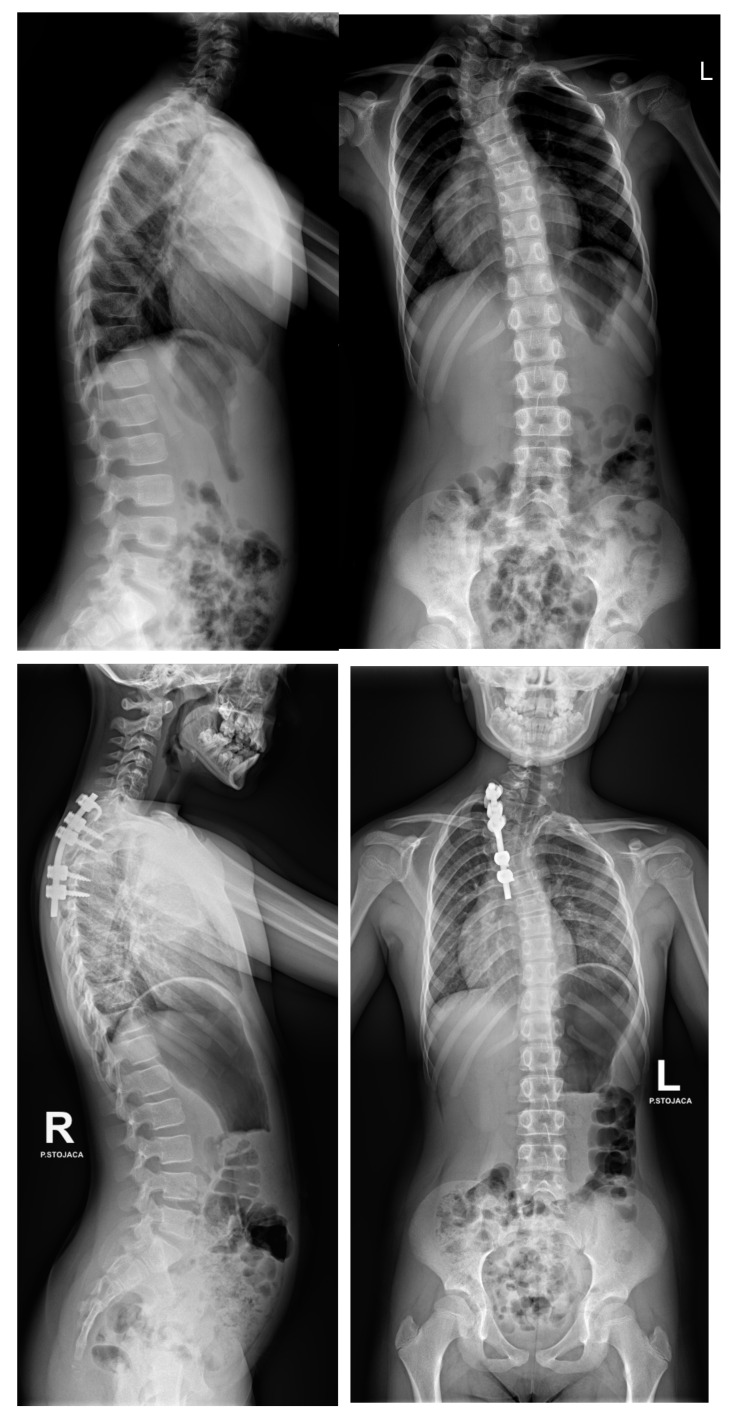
A 6-year-old girl with congenital scoliosis and kyphosis was treated with in situ arthrodesis due to multi-level segmentation defects in the spine. Given the complexity of the anomalies, this treatment technique was considered the least risky and provided satisfactory outcomes. X-rays are shown from before surgery and at the 4-year follow-up.
